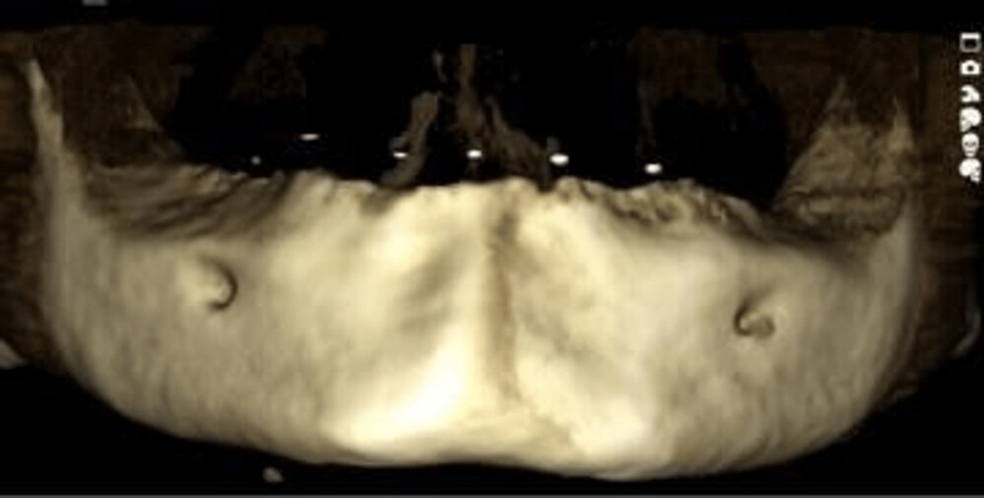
CBCT scan and 3D imaging of the edentulous mandible after placing the stent with embedded gutta-percha cones at prospective implant sites. The gutta-percha cones can be visualised on the edentulous mandible as shown in the figure. CBCT: cone beam computed tomography.
